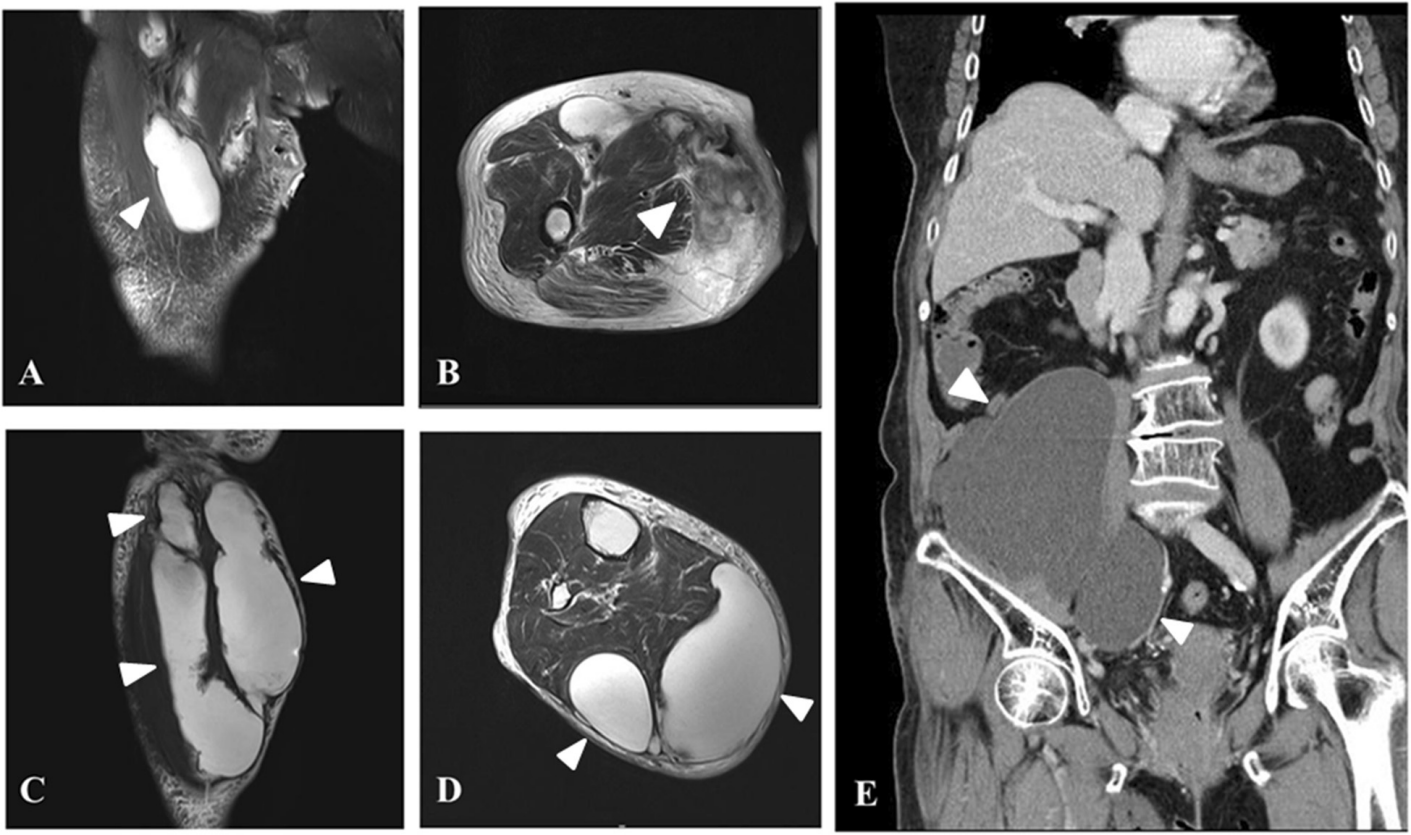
Image findings. a A T2-weighted coronal view magnetic resonance image (MRI) of the right thigh shows a cystic lesion mainly involving the anterior compartment, especially the sartorius muscle (an *arrowhead*). b A T2-weighted axial view MRI reveals lesions mainly involving the medial compartment along the fascia of the hamstring muscles (an *arrowhead*). c A T2-weighted coronal view of MRI of the right calf shows multiloculated and lobulated lesions mainly involving the posterior compartment (*arrowheads*). d A T2-weighted axial view MRI reveals a lesion along the superficial fascia (*arrowheads*). e A coronal view abdominal computed tomography scan shows a large multiloculated cystic mass occupying the right retroperitoneal space (*arrowheads*)
